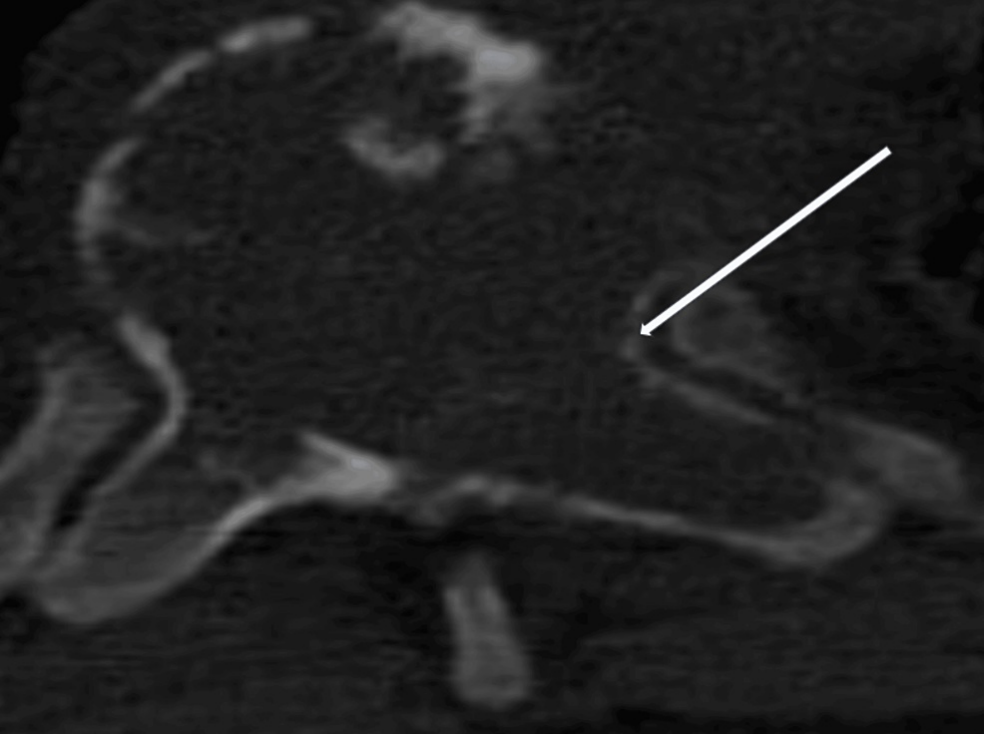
Computed tomography (CT) of the spine axial view, T8 lytic lesion involving the vertebral body, extending into the pedicle (arrow) and lamina
